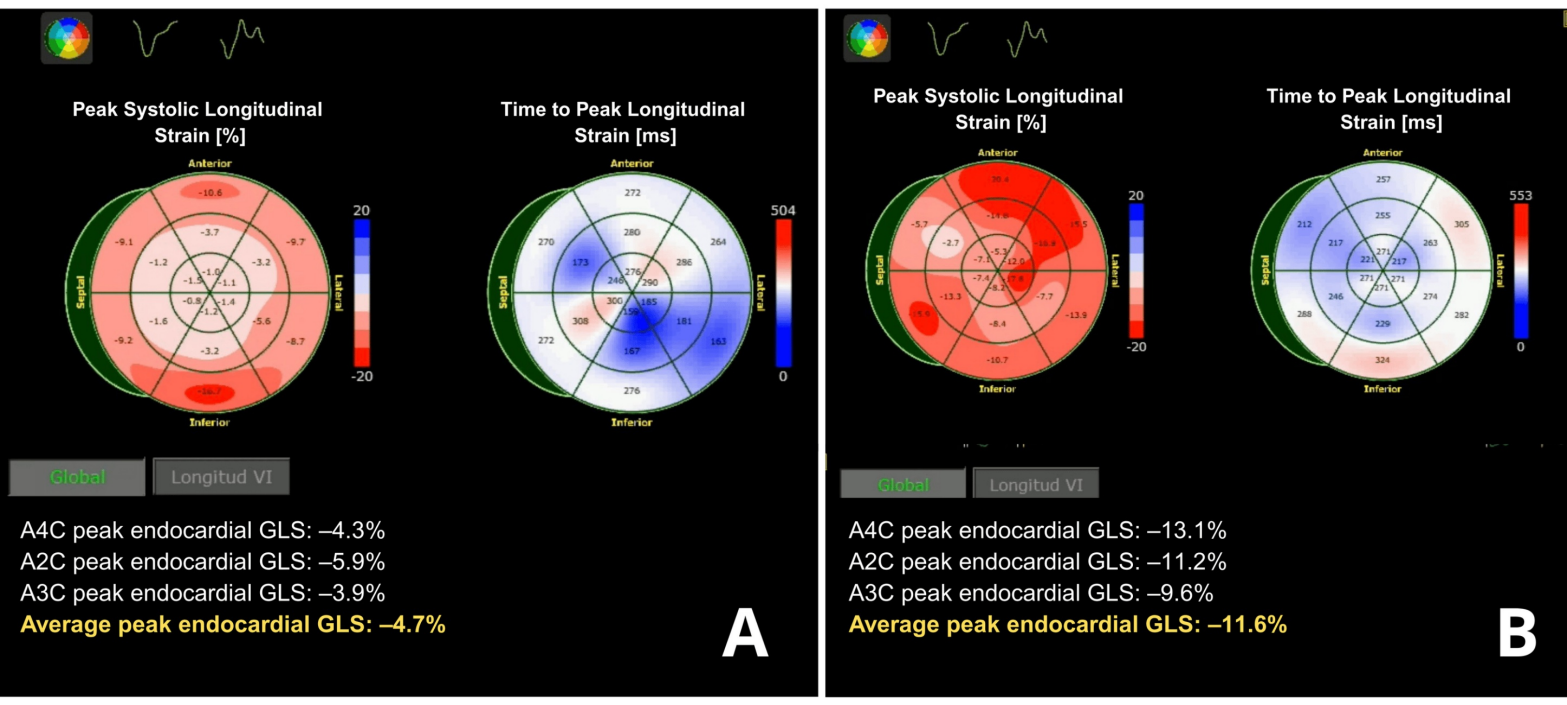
Evolution of global longitudinal strain demonstrating severe initial myocardial dysfunction and subsequent recovery (A) Baseline speckle-tracking echocardiography obtained on admission demonstrating severely reduced peak endocardial global longitudinal strain (GLS), with an average GLS of –4.7%, consistent with severe acute myocardial dysfunction. (B) Follow-up speckle-tracking echocardiography demonstrating marked improvement in myocardial deformation parameters, with recovery of peak endocardial GLS to an average of –11.3%, reflecting substantial functional myocardial recovery following mechanical circulatory support and clinical stabilization.
